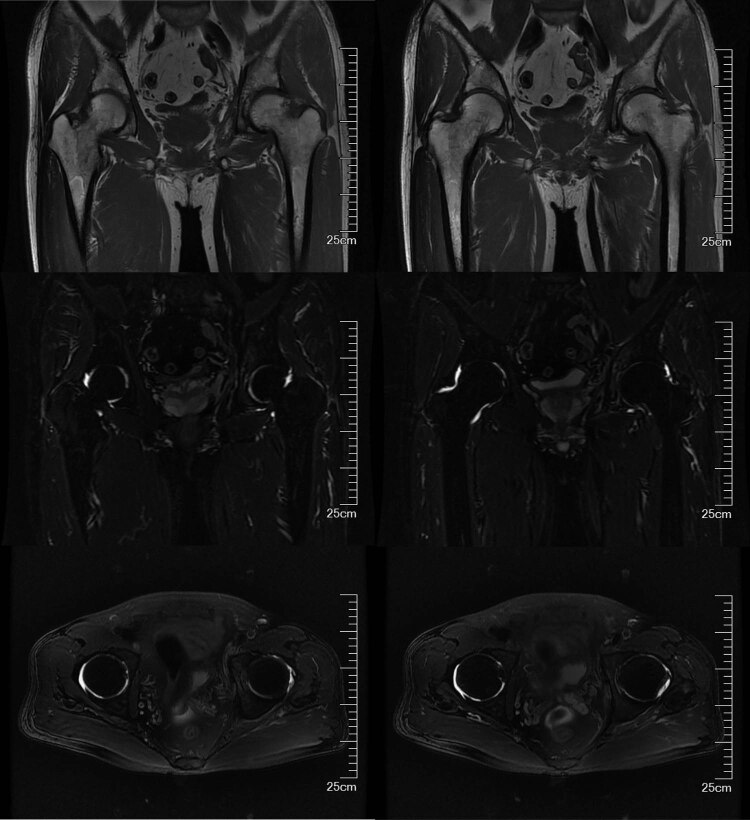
MRI of the hip joint shows a patchy hyperdense focus in the left acetabulum, a small amount of fluid signal shadow in the bilateral acetabulum, and no obvious swelling in the surrounding soft tissues.
